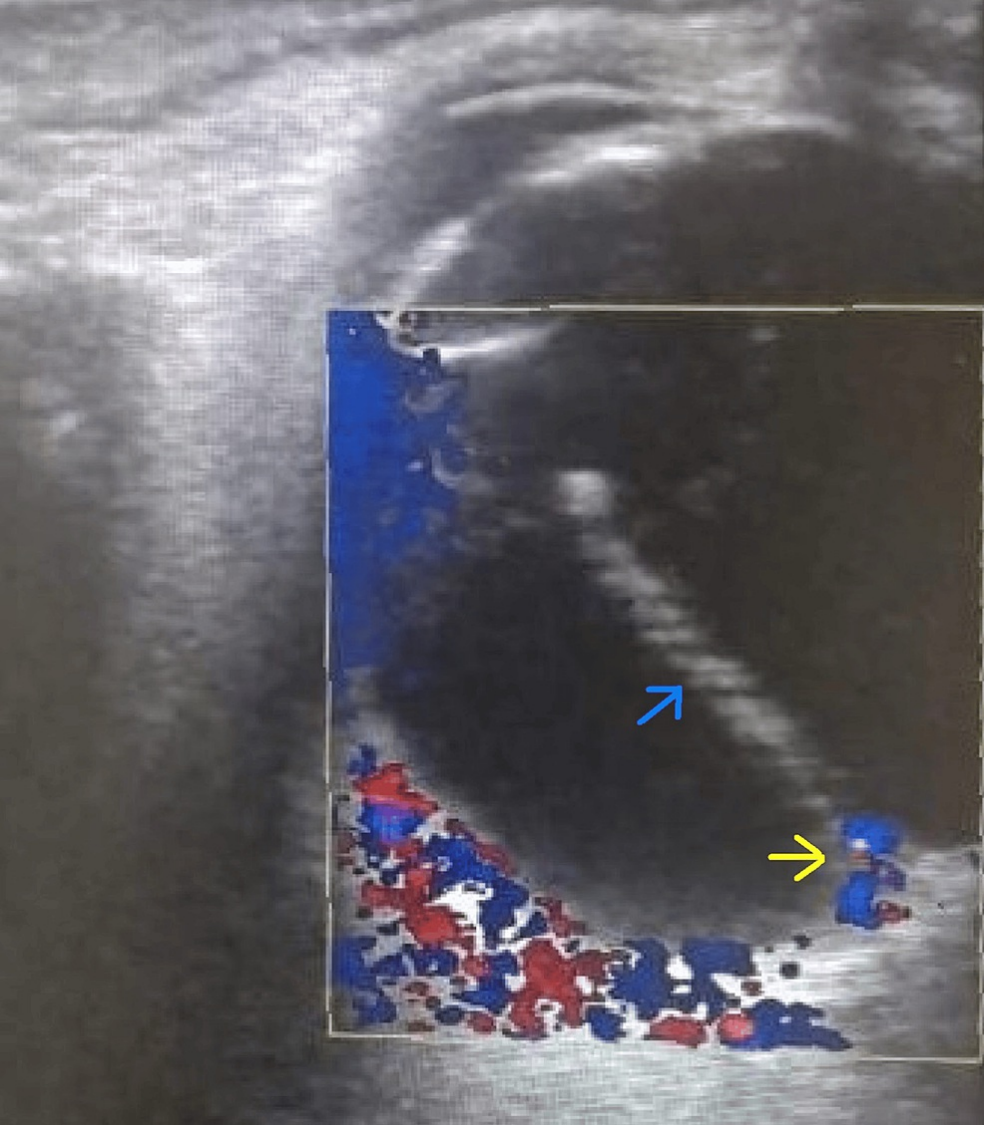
Ultrasound colour doppler of right orbit demonstrating vascular stalk running from optic disc to anterior vitreous phase(blue arrow) with corresponding internal vascularity in posterior third segment(yellow arrow) representing persistent fetal vasculature.
